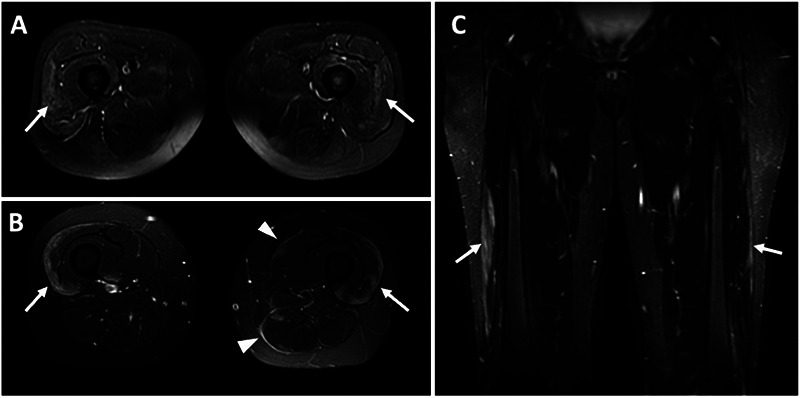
Magnetic resonance imaging in inflammatory myopathies. A) Axial STIR-weighted imaging of a patient with polymyositis with hyperintense areas of muscular edema, mainly in quadriceps (arrows). B) Axial STIR-weighted in a patient with dermatomyositis demonstrating a perifascial hyperintensity (arrows) and well-demarcated fascial hyperintense signal indicating fasciitis (arrowhead). C) Coronal STIR-weighted imaging with peripheral hyperintensity (arrows) in a patient with dermatomyositis.
